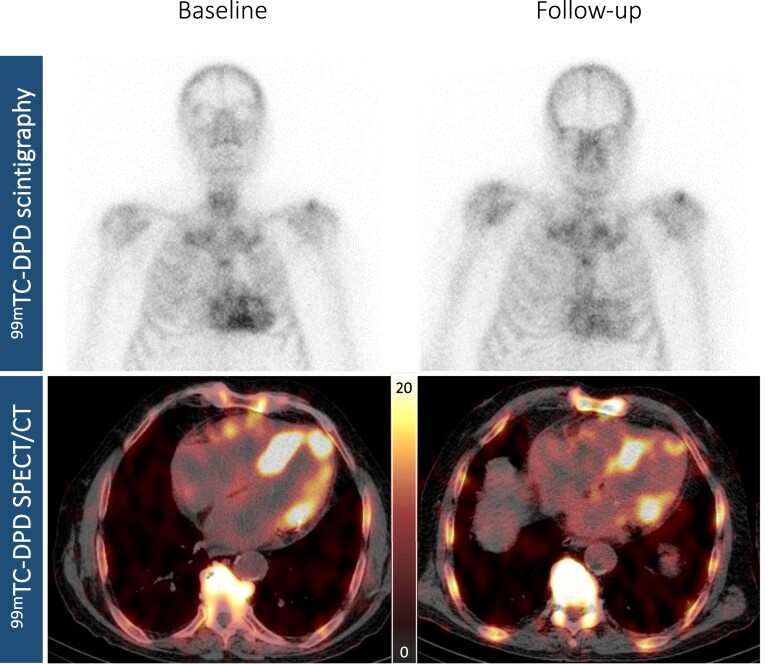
Visualization of response to tafamidis treatment by nuclear imaging. Planar whole-body ^99m^Tc-3,3-diphosphono-1,2-propanodicarboxylic acid (DPD) image (upper panels) and axial ^99m^Tc-DPD single-photon emission computed tomography/computed tomography (SPECT/CT) imaging (lower panels) of a representative tafamidis treatment-responsive patient with wild-type ATTR-CM at baseline (left panels, Perugini grade 3, SUV peak cardiac: 12.40 g/mL) and at follow-up (right panels, Perugini grade 2, SUV peak cardiac: 8.56 g/mL).
